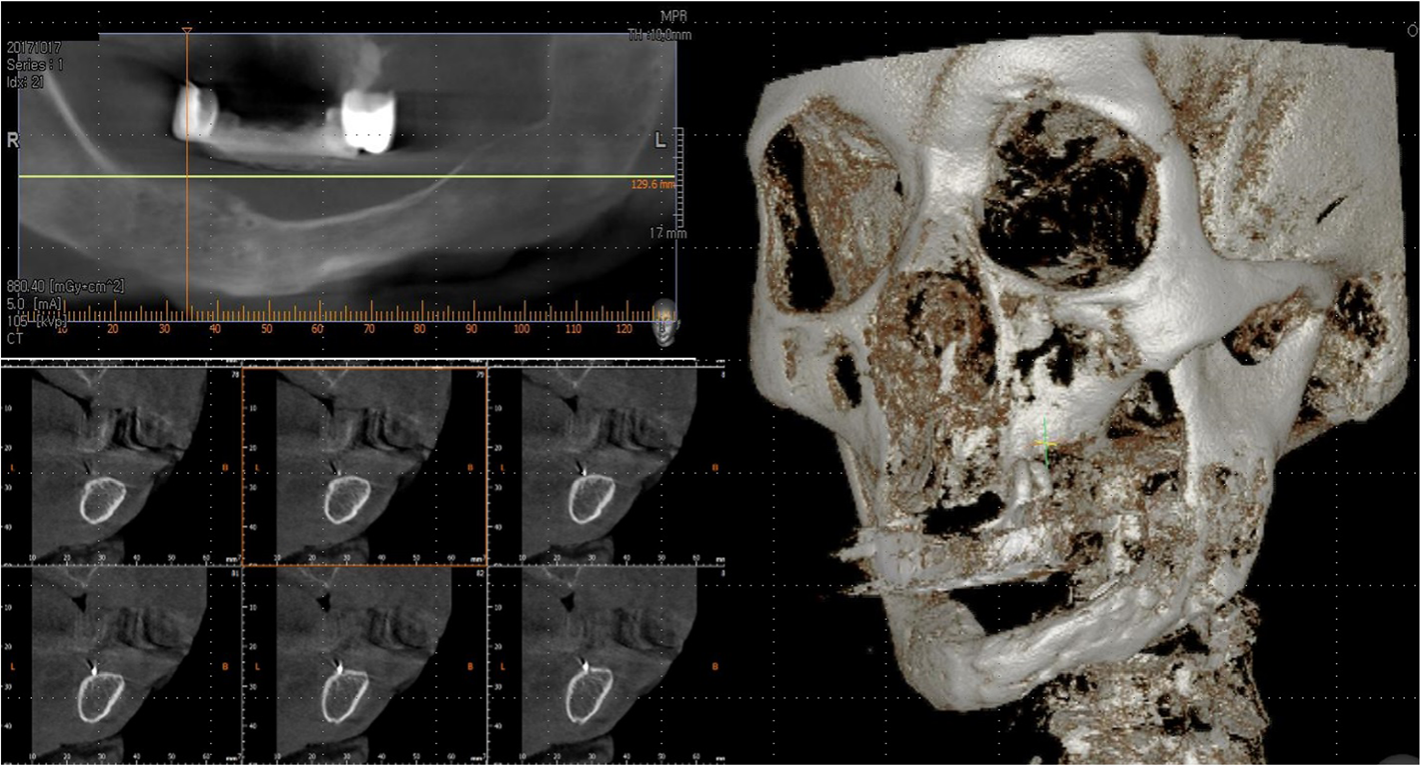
Neomandible body on CT view
